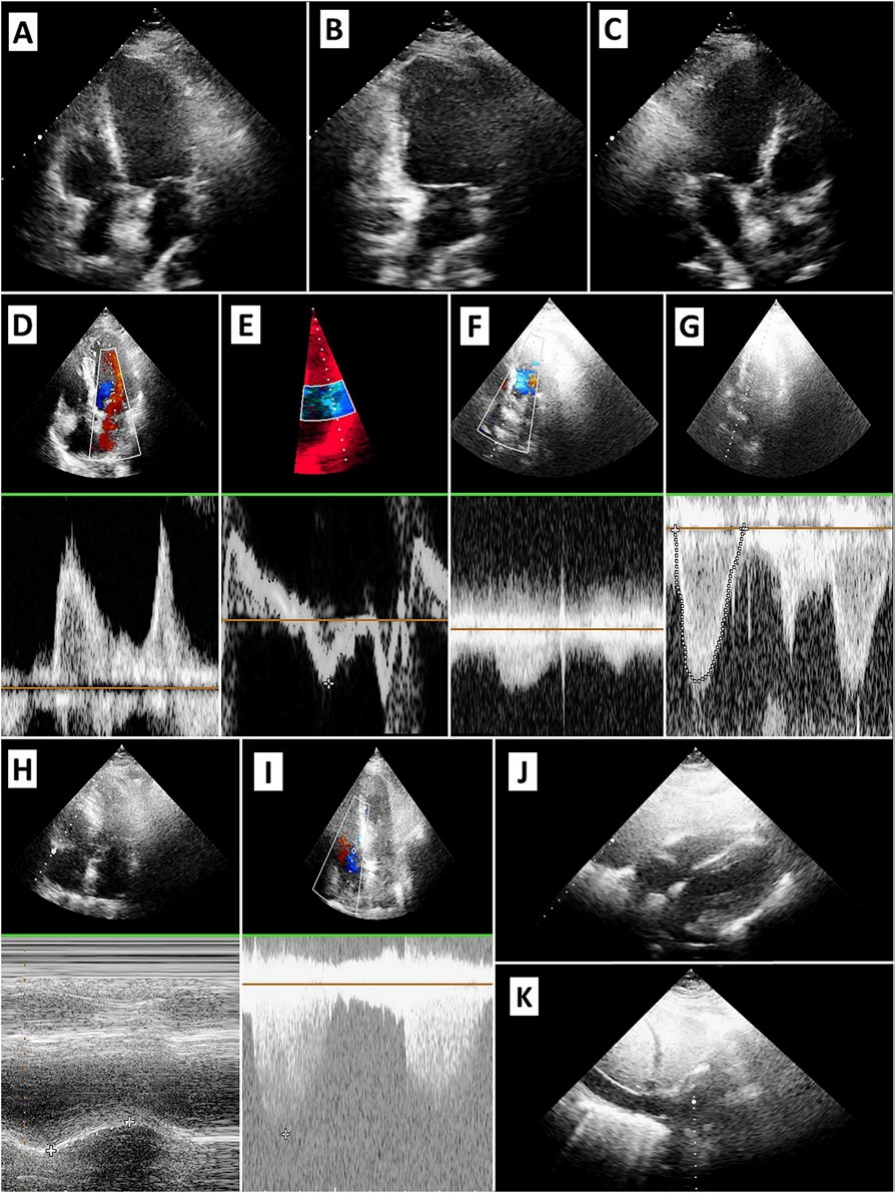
Showing the images obtained by apical-subcostal protocol. A: Apical Four-chamber. B: Apical Two-chamber. C: Apical three-chamber. D: Mitral Inflow by Pulsed-wave Doppler (E and A wave). E: Pulsed Tissue Doppler of the Lateral Mitral Valve Annulus. F: Aortic Flow by Continuous Doppler. G: LV Outflow Tract VTI. H: TAPSE. I: Gradient Insufficient Tricuspid. J: Subcostal Cardiac Chamber. K: Inferior Vena Cava
